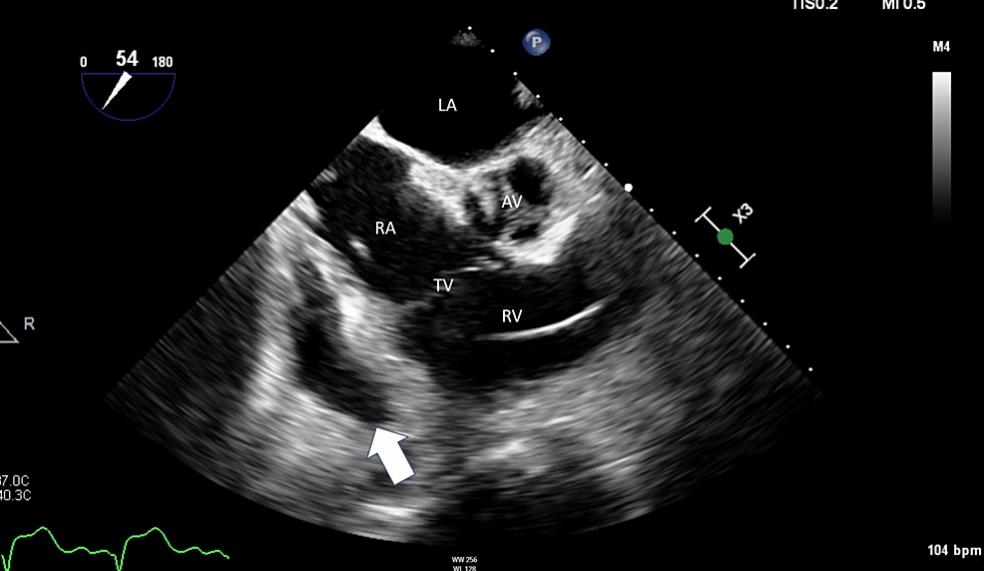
Two-dimensional TEE still frame showing the mid esophageal aortic valve short axis view (54°) during early systole, demonstrating a loculated pericardial effusion (arrow) adjacent to the RA and RV TEE: Transesophageal echo; AV: Aortic valve; LA: Left atrium; RA: Right atrium; RV: Right ventricle; TV: Tricuspid valve
